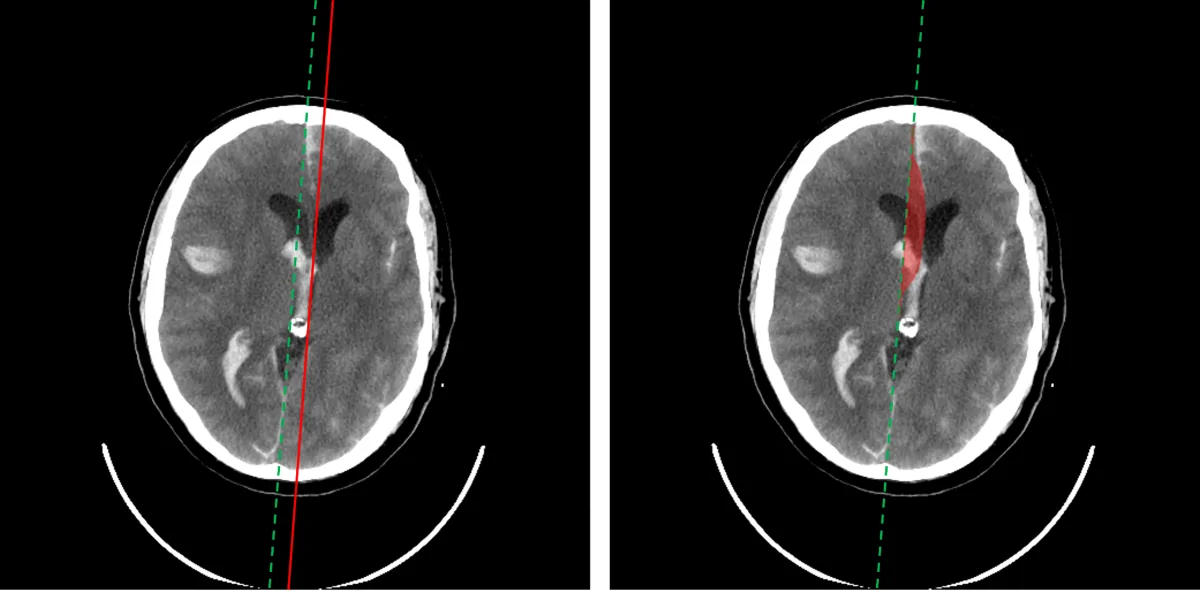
Visual comparison of automated linear midline shift and volumetric midsurface shift estimation techniques applied to a single noncontrast head computed tomography scan of a patient with traumatic brain injury. Adapted from Jiang et al [104], which is published under Creative Commons Attribution 4.0 International License [111], using a sample from the CQ500 dataset.
